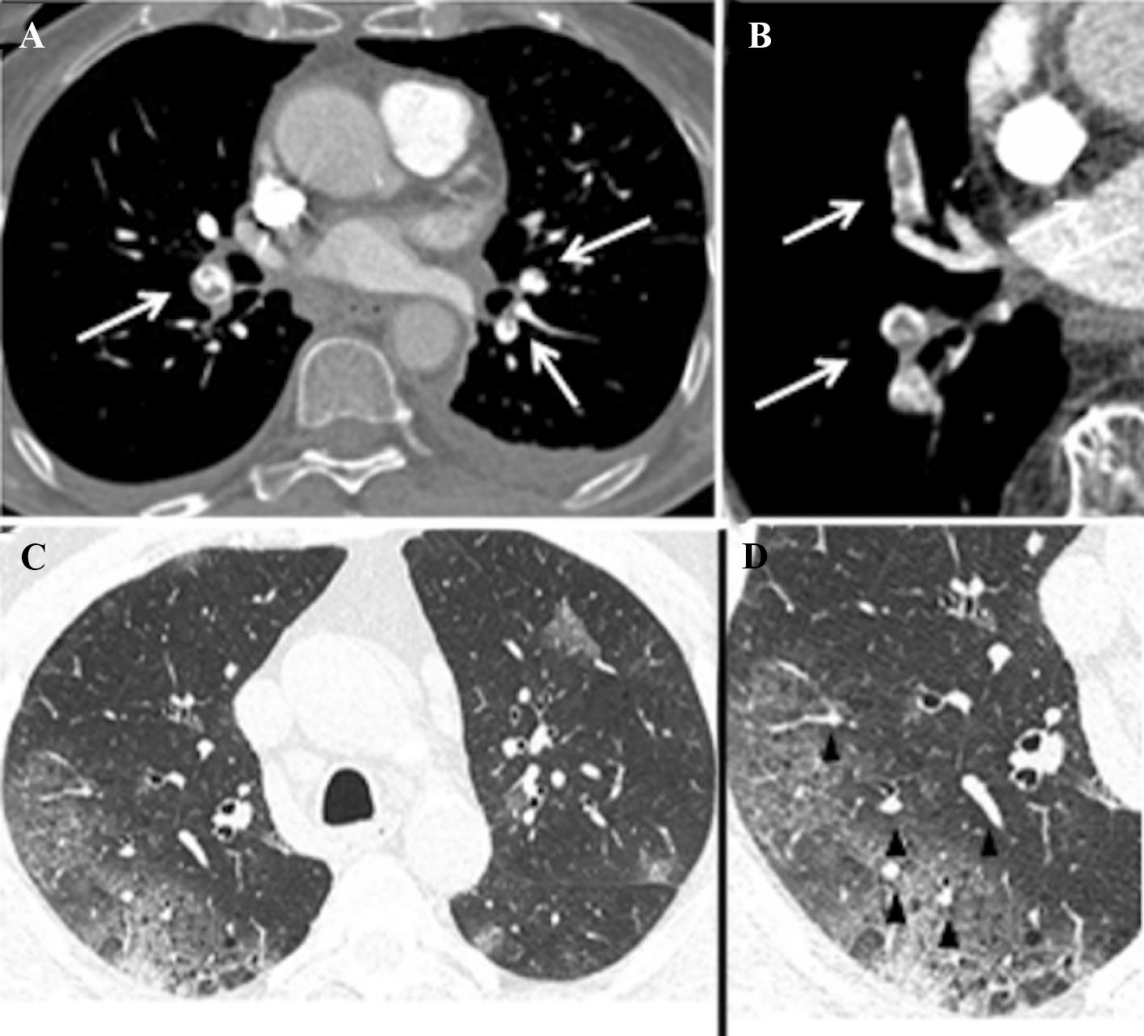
Pulmonary CT angiography of a 78-year-old male obtained 17 days after the onset of COVID-19 symptoms. A, B Axial contrast-enhanced CT scan shows bilateral filling defects involving lobar branches of the pulmonary artery (white arrows). C, D, Axial CT images (lung window) show peripheral ground-glass opacities with associated areas of consolidation, lung architectural distortion and vascular enlargement (black arrowheads)
